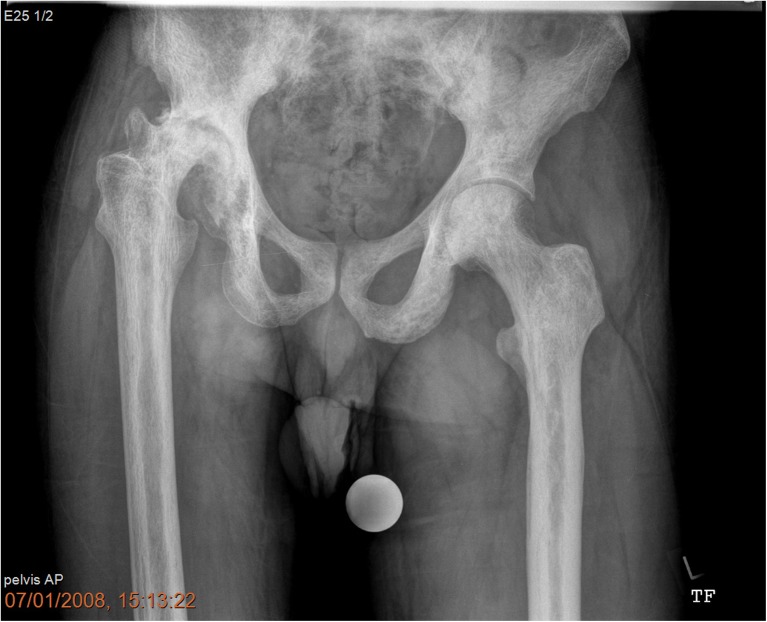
Pre-operative radiographs of a 48-year-old male, with Steinberg stage VI osteonecrosis right hip with “femur within femur” appearance
